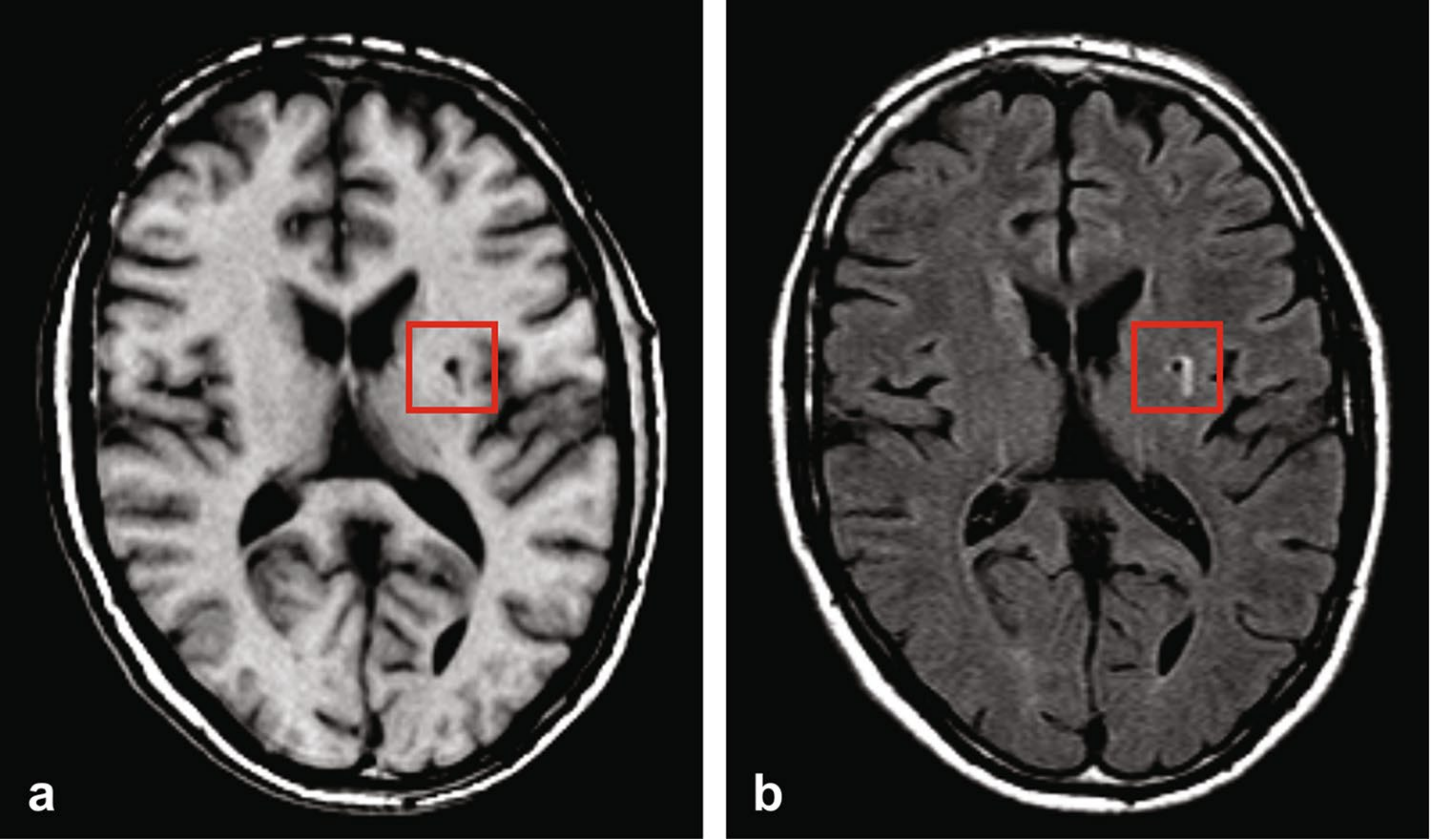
Example transversal image slice of the T1-weighted (a) and T2-FLAIR (b) acquisitions. A brain infarct can be observed in the right hemisphere next to the basal ganglia, in the red square, as the hypointense region in the T1-weighted image (a) and the hypointense region with hyperintense ring in the T2-FLAIR image (b).
